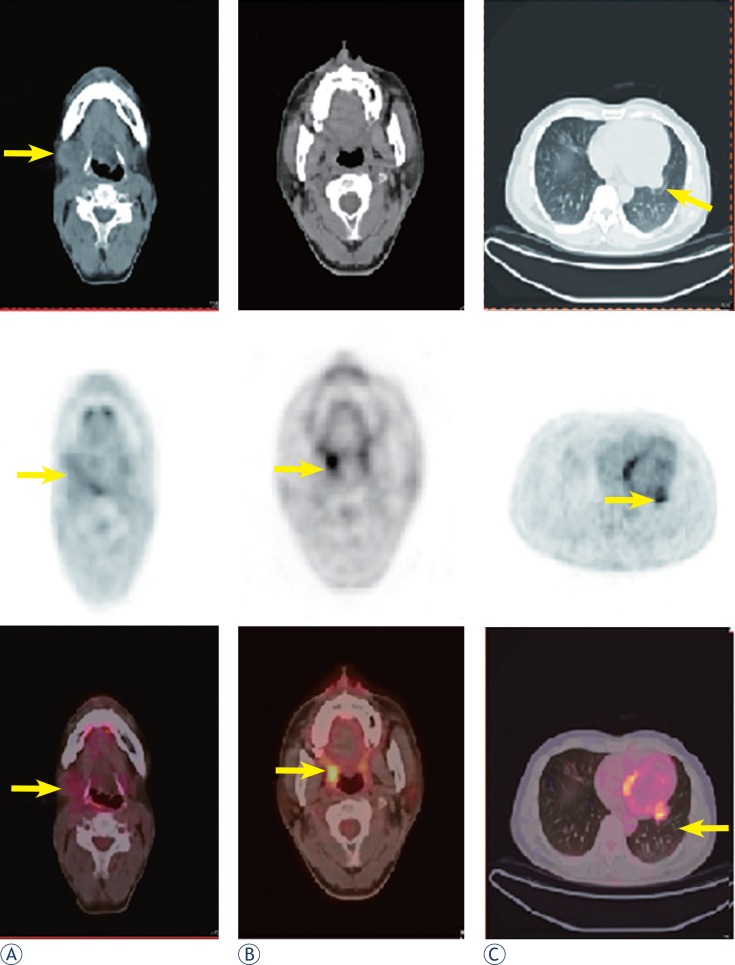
(a, b) Nine months after the second surgery, PET/CT revealed high FDG uptake in the right submaxillary lymph node (SUV_max_= 3.2), right oropharynx (SUV_max_= 5.4) and(c) multiple nodules in both lungs (SUV_max_= 4.6).
